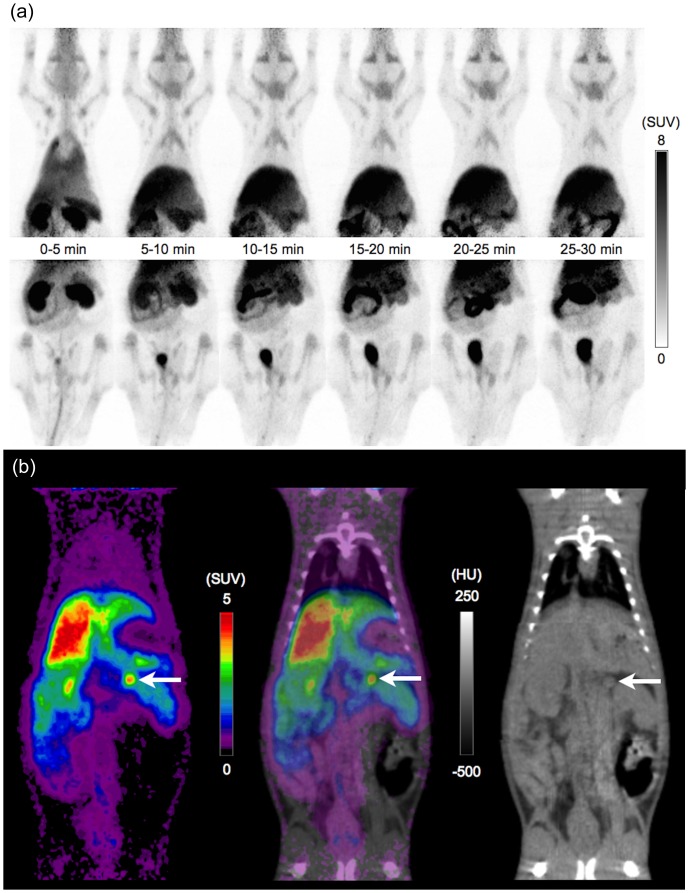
Whole body images after the administration of ^11^C-DNP: (a) dynamic maximum intensity projection images of PET, (b) coronal images of PET, CT, and PET/CT (20–40 min, arrow: left adrenal gland).
